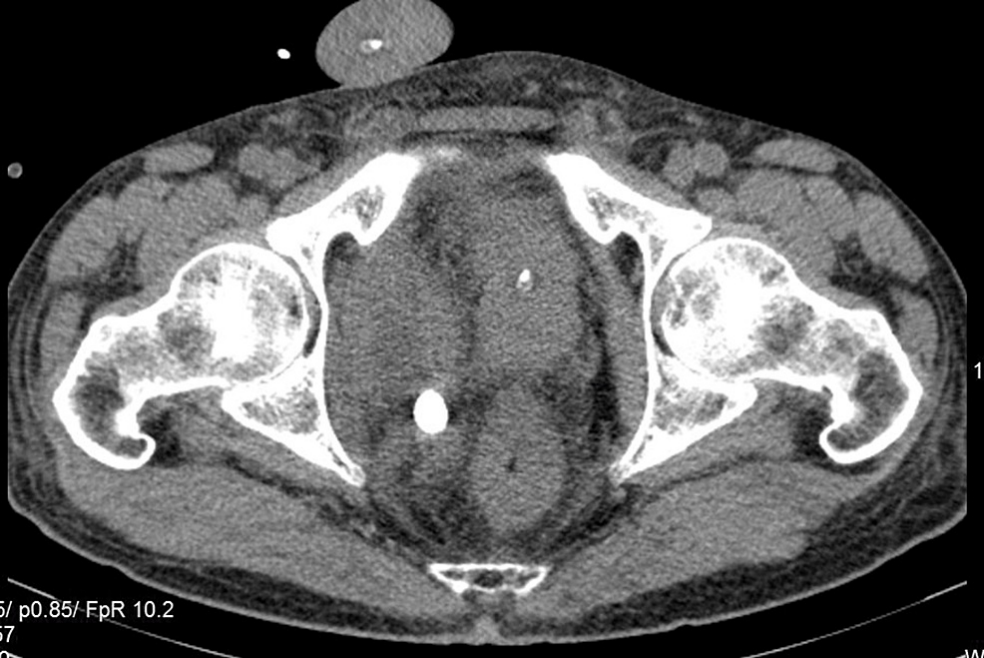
Axial plane of contrast CT scan depicting extravesical calculus. CT, computed tomography
